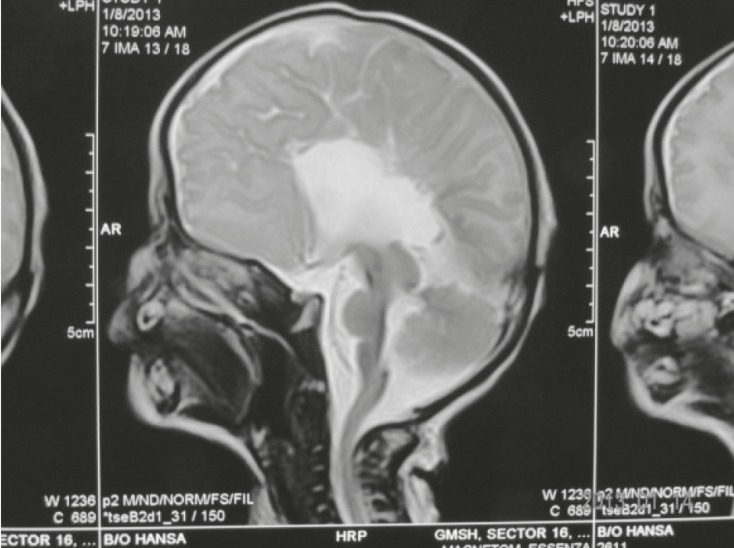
Coronal MRI showing corpus callosum Agenesis
